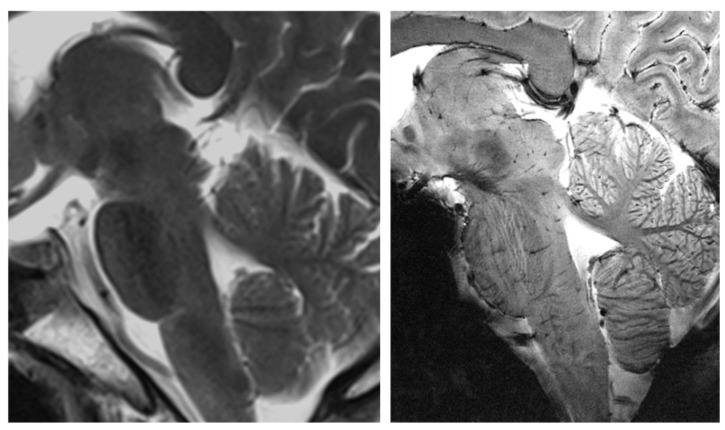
Comparison of 3.0T (left) and 7.0T (right) MRI in the brainstem area. As shown above, markedly different detail is visualized on the 7.0T MRI, such as the details of the thalamic and brainstem areas, including the midbrain and pons. Reprinted with permission from Cho et al., 2014, Elsevier [13].
